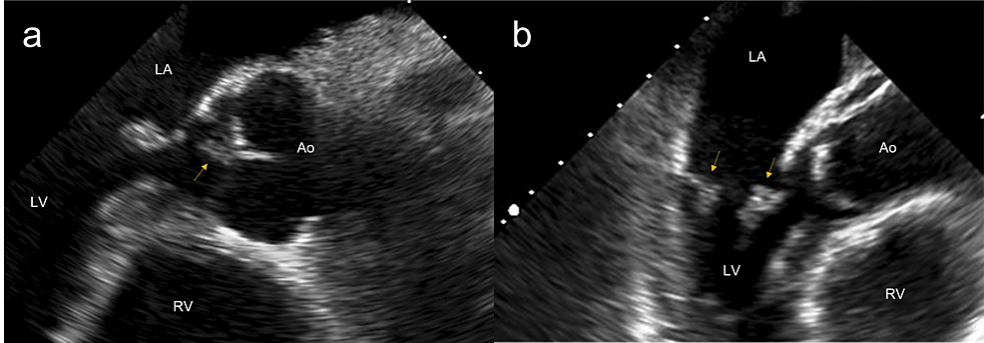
Trans-esophageal echocardiogram. (a) Mid-esophageal long-axis view with a vegetation on the atrial aspect of the aortic valve (arrow). (b) Mid-esophageal 3-chamber view with vegetations on both leaflets of the mitral valve (arrows). LA: left atrium; LV: left ventricle; RV: right ventricle; Ao: aorta.
